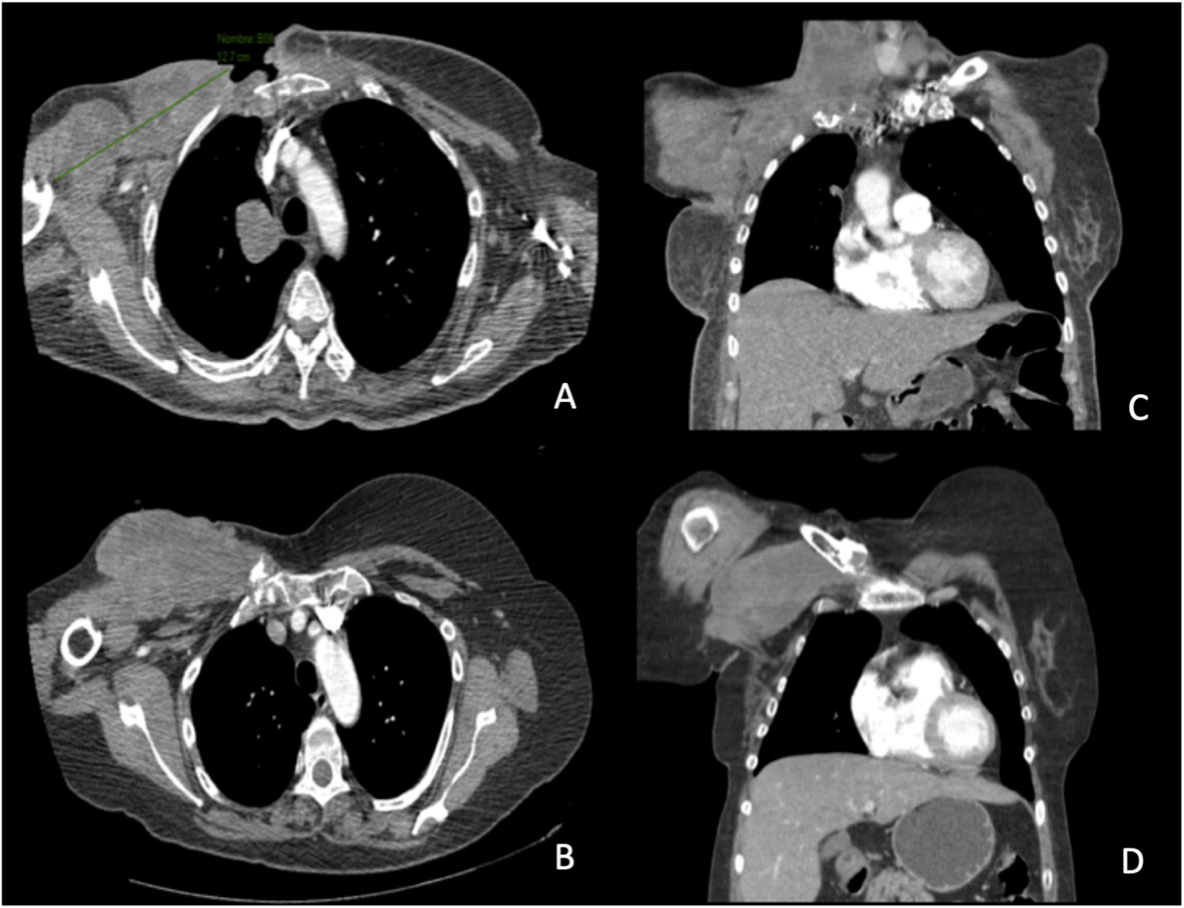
Thoracic computed tomography in axial (a, b) and coronal (c, d) projections of two of the patients, showing an infraclavicular mass appearance on the thoracic wall and axillary extension, all coinciding with the radiation fields
